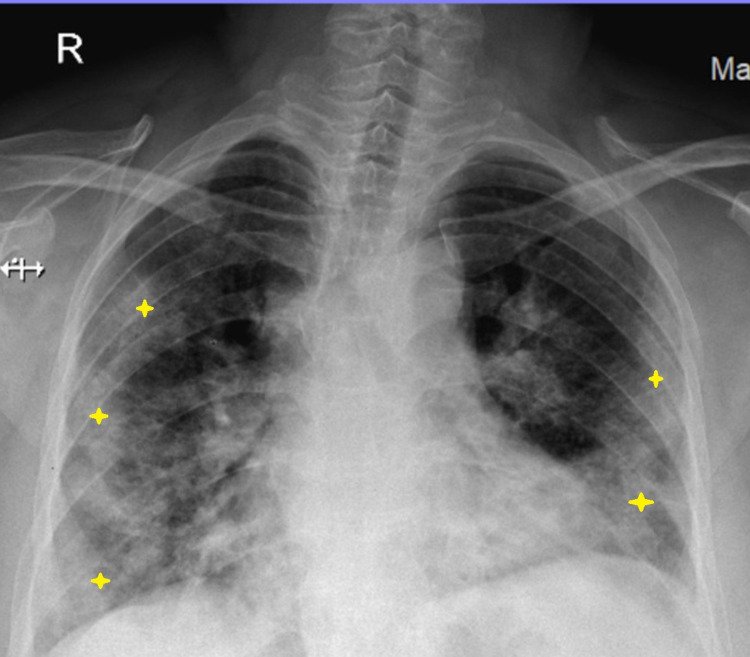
Chest X-ray showing bilateral radio-opacities suspicious for COVID-19 pneumonia. COVID-19: coronavirus disease 2019
